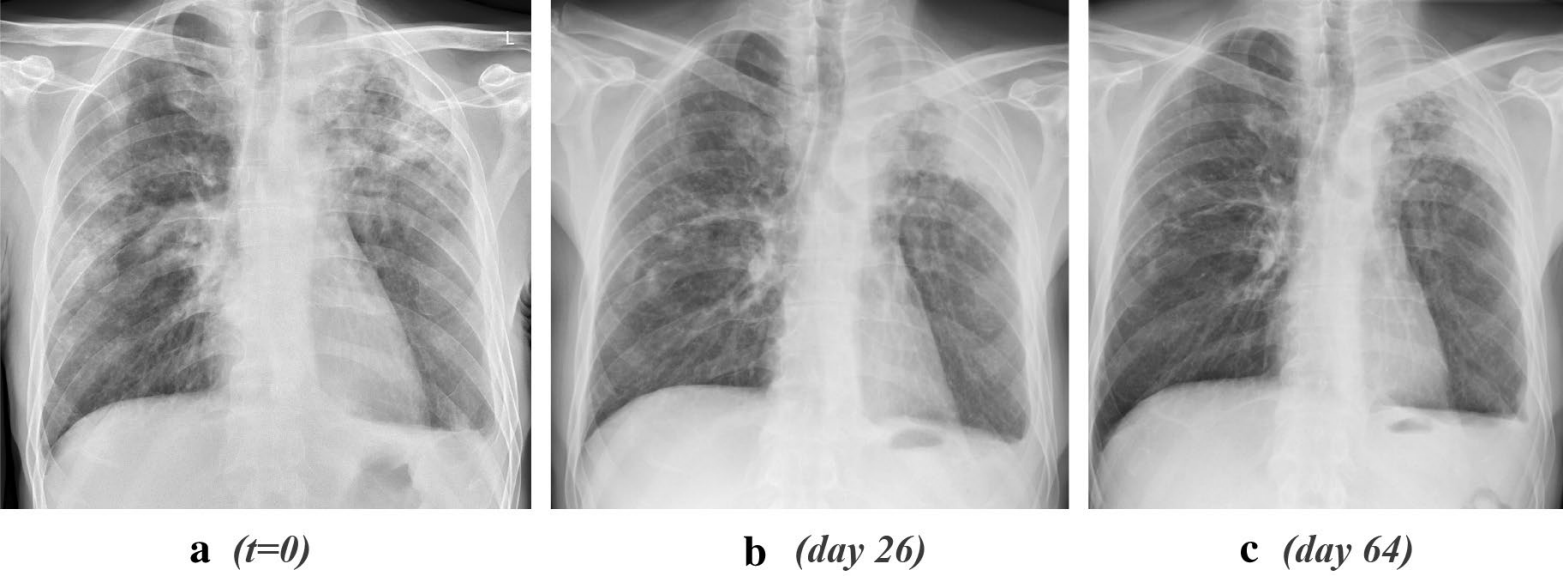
a–c Chest X-ray comparison of patient B on day 1(left), day 26 (middle) and day 64 (right). This sequence of radiographs shows an increase of infiltrative consolidation and pleural thickening in the LUL, corresponding to the manifestation of PR symptoms and a subsequent reversal of these changes (c) following corticosteroid therapy initiation
